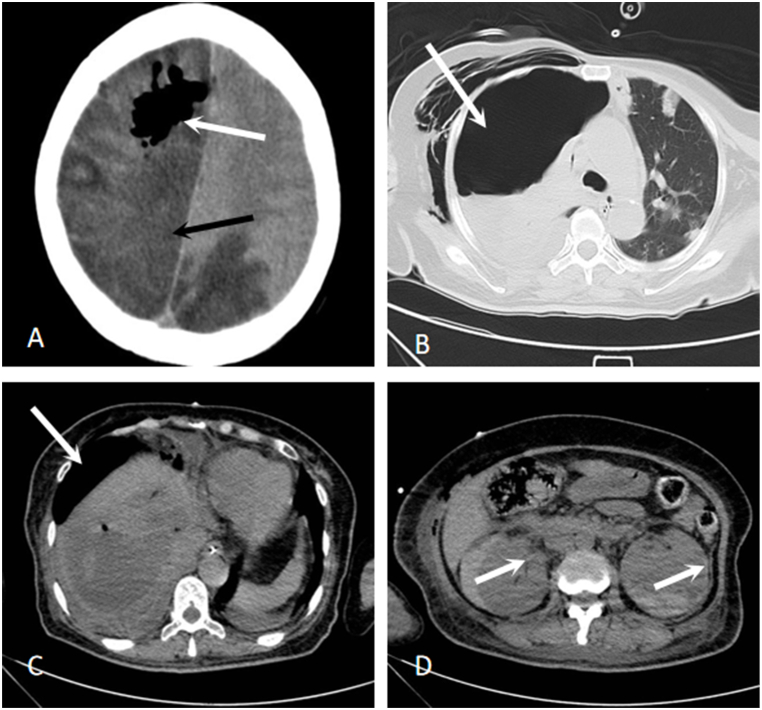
Axial CT images: (A) Extensive infarction (black arrow) with multiple gas accumulations (white arrow) and formation of cerebral herniation in the right cerebral hemisphere and left parietal and occipital lobes; (B) Right lung showing pneumothorax with lung consolidation and atelectasis, subcutaneous emphysema in the neck and chest (white arrow); (C) Decreased size of the enormous lesion in the liver, with retained drainage tube (white arrow); (D) Minor hemorrhagic foci in both kidneys (white arrow).
